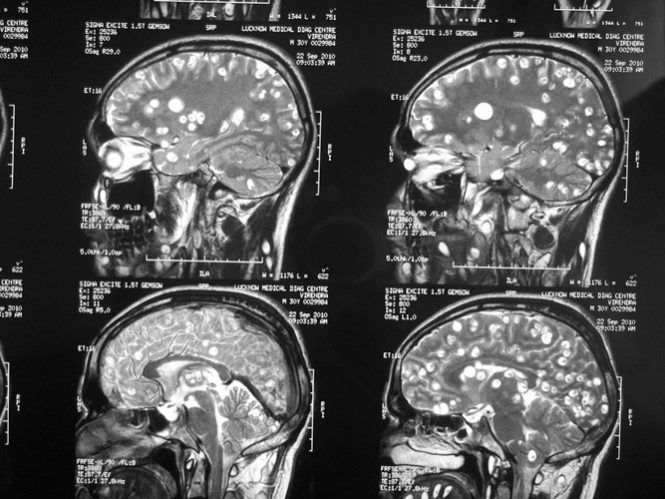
Magnetic resonance imaging of the cranium, T2 weighted sagittal image showing multiple well-circumscribed cysts with presence of scolex.
